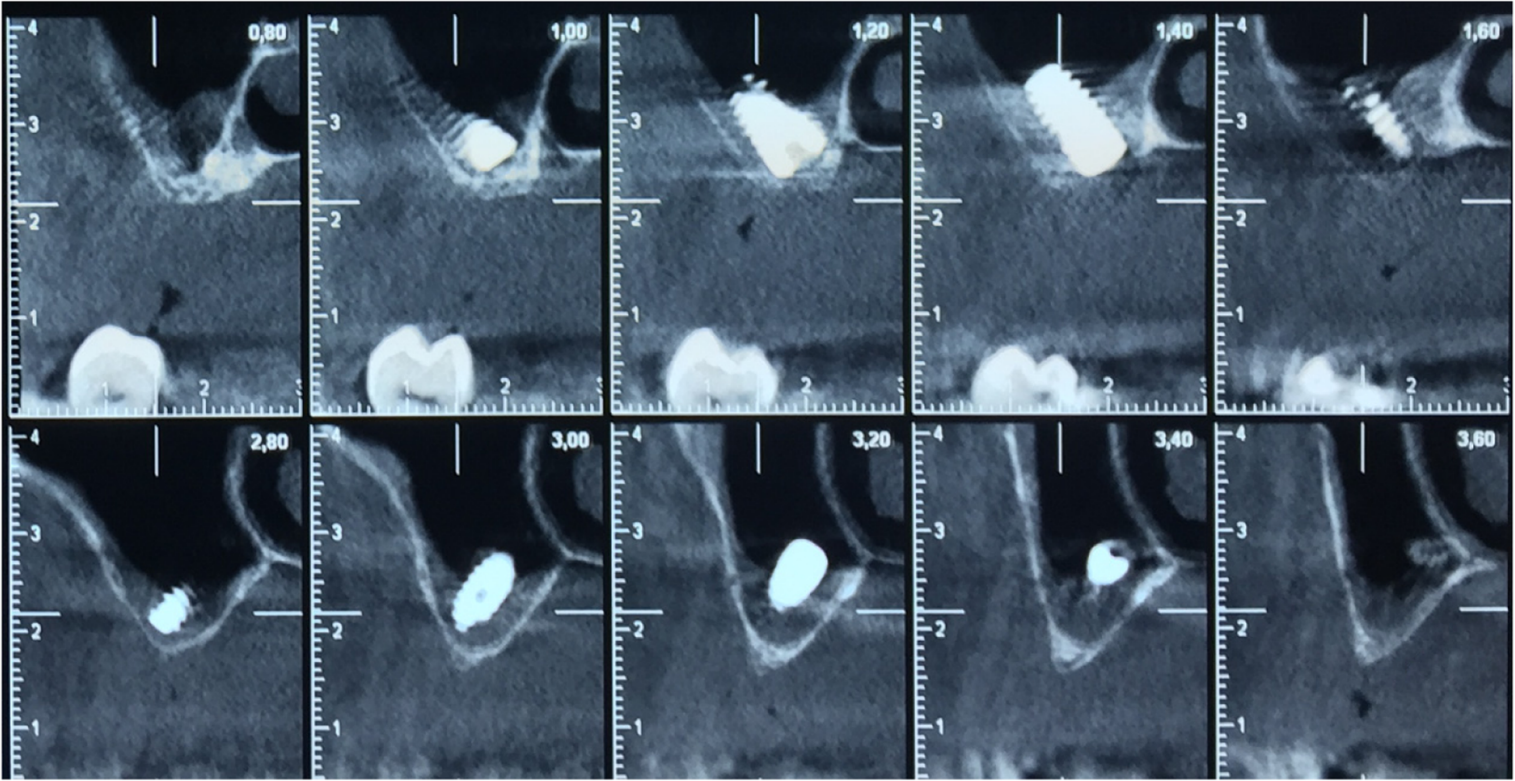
Revelation of the exact position of both implants inside the right maxillary sinus with a CBCT
